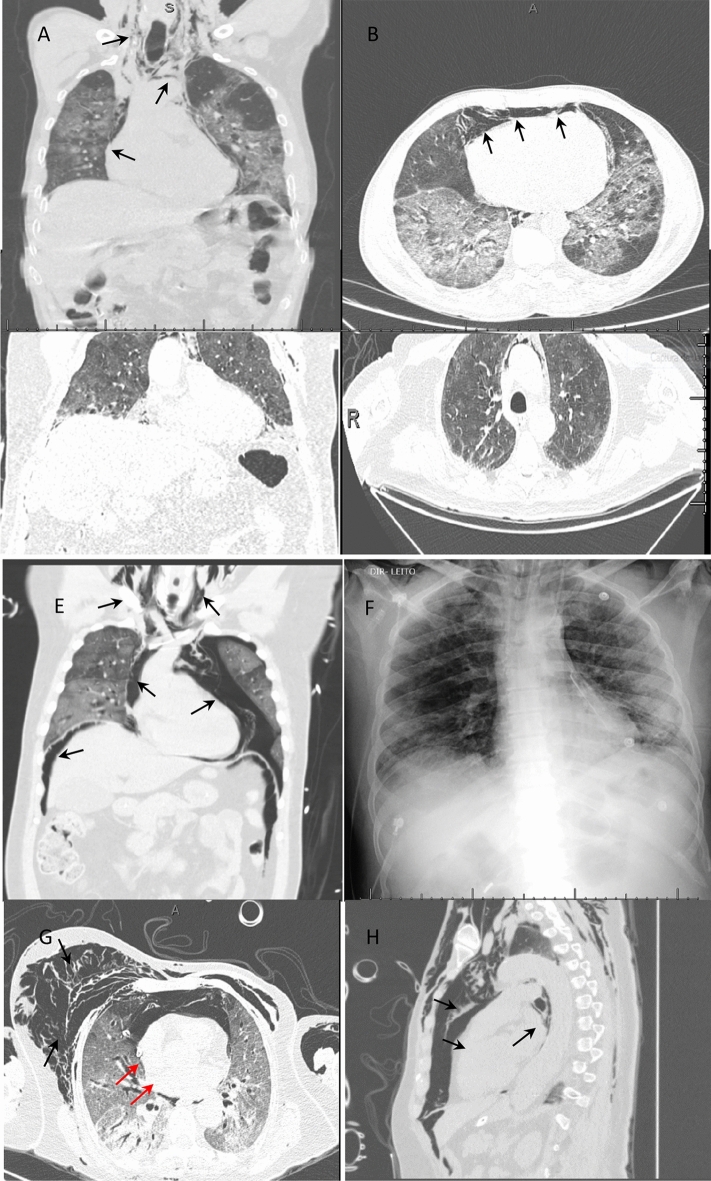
Chest CT scans or radiograph of the chest showing pneumomediastinum (black arrows). A, B Case 1. C, D Case 2. E, F Case 3. G, H Case 4. Red arrows: chest drainage
